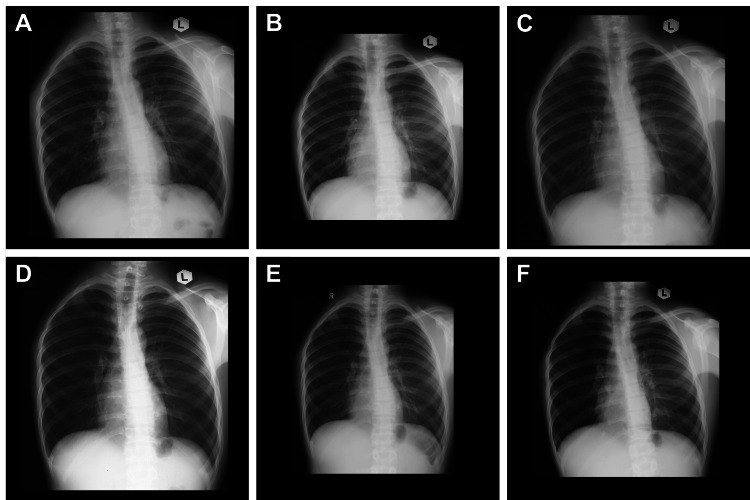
Follow-up chest X-rays for surveillance, taken at (A) one month, (B) four months, (C) seven months, (D) 10 months, (E) 12 months, and (F) 17 months after end of treatment. No evidence of pulmonary metastases
